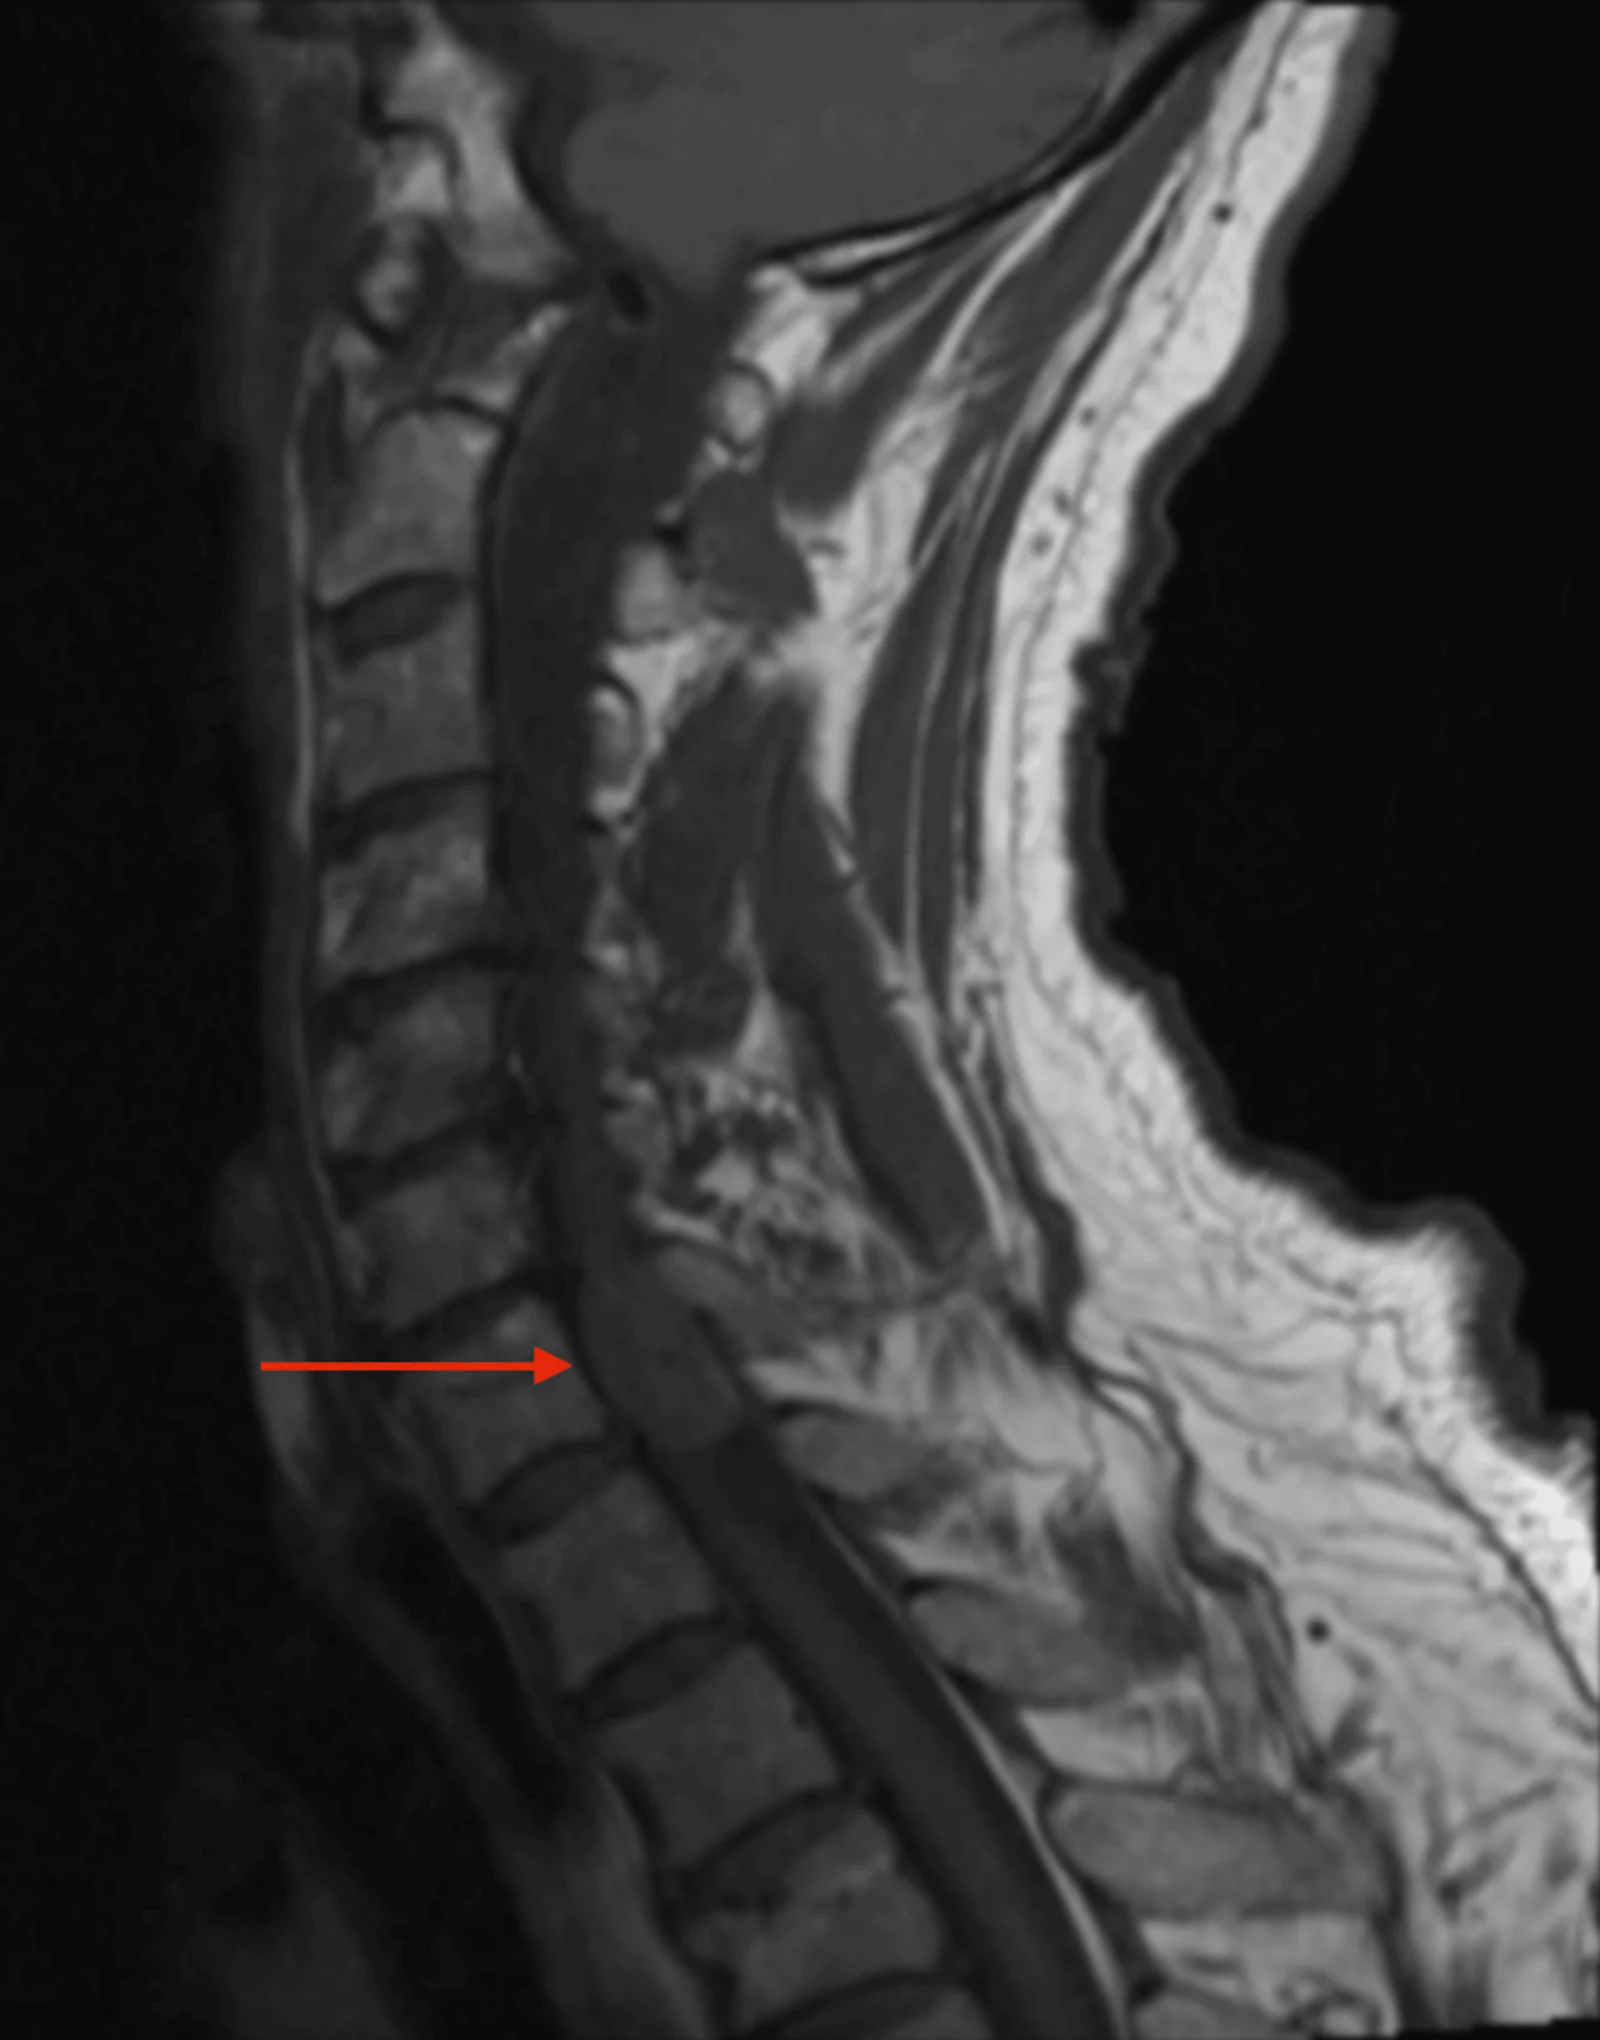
T1 without contrast spinal MRI Sagittal T1-weighted cervical spine MRI without contrast demonstrating an enhancing intradural extramedullary mass consistent with a meningioma, producing focal spinal cord compression.
